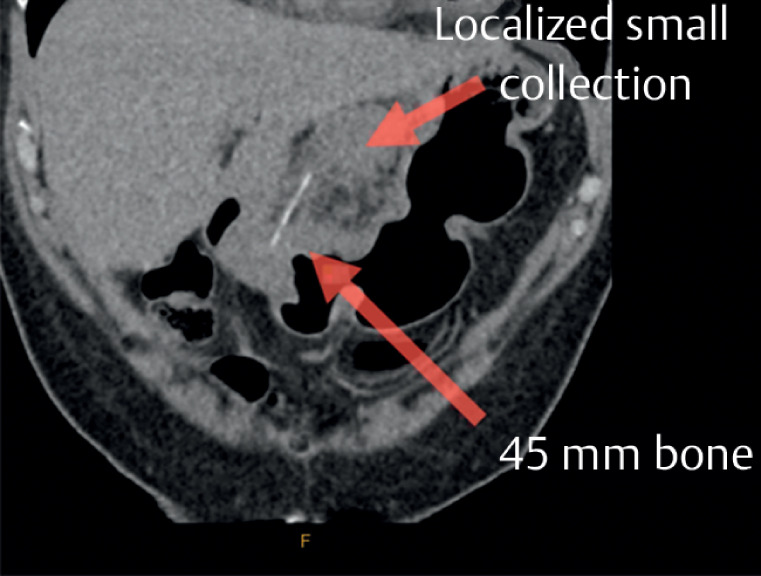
Computed tomography showing a 45-mm foreign body lodged in the distal stomach, with a 17 × 14 mm collection.
